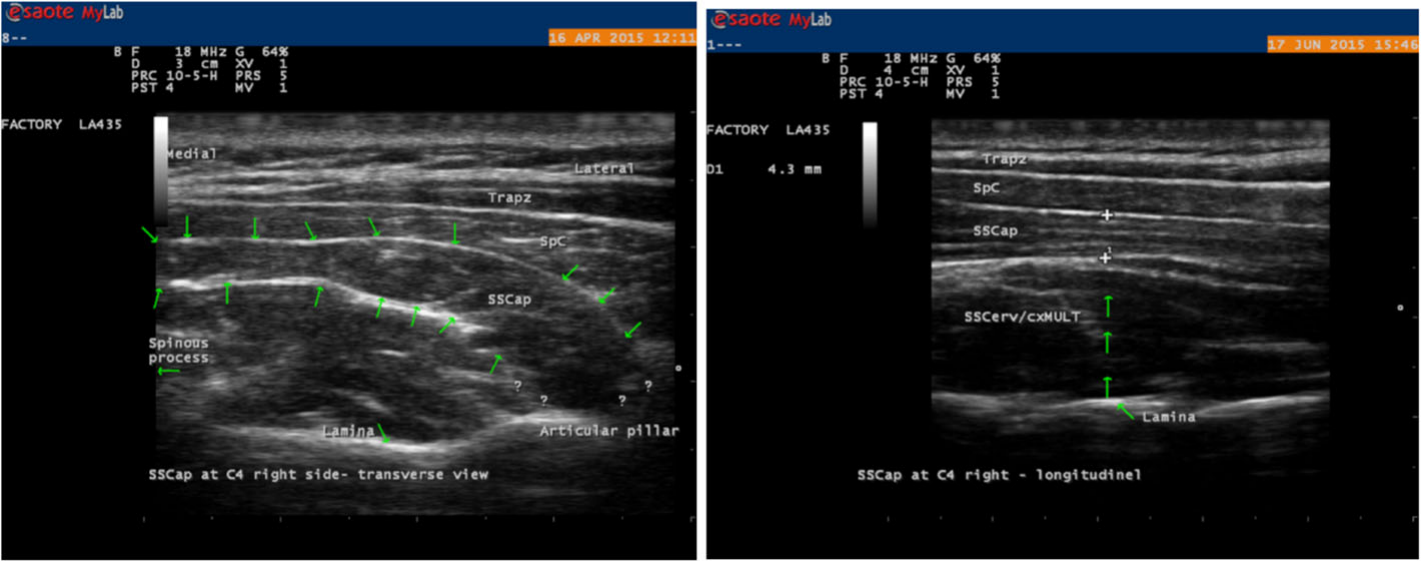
Ultrasound image of the Sscap at C4 in transverse view to the left for dynamic visualisation of anatomical borders and in longitudinal view where measurements were taken to the right. The caliper is placed from the lower border the Sscap against the Dce above the midpoint of the lamina of C4 to the outer border of Sscap against the Splenius Capitis muscle
